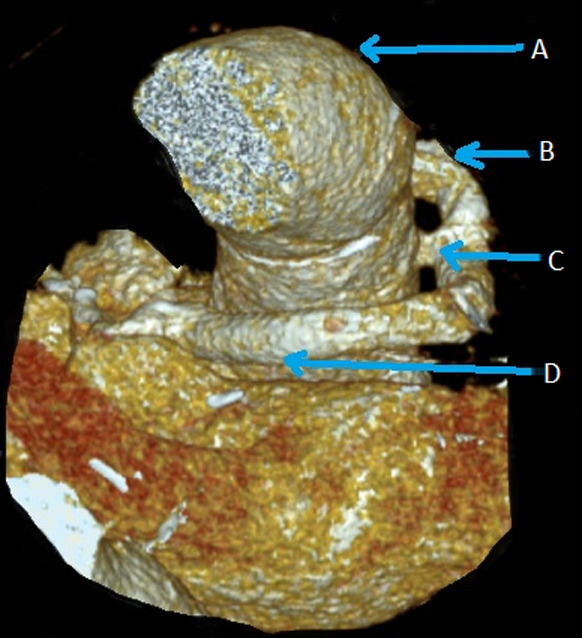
post-operative computed tomography angiography scan showing excellent surgical resultants: A) ascending aorta; B) right coronary tube; C) T-suture after redo; D) left coronary tube
